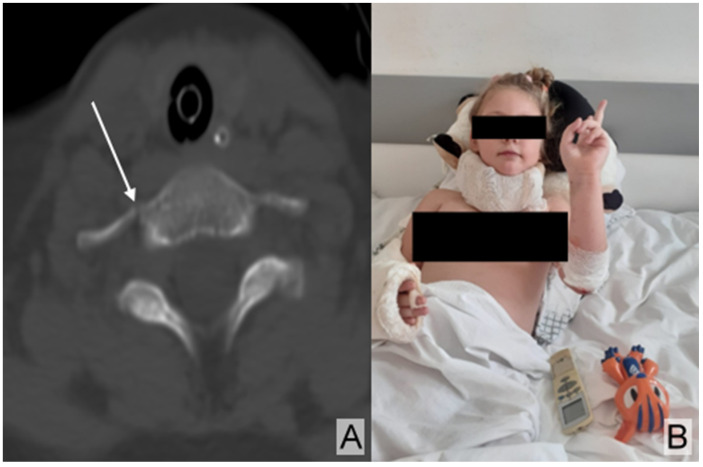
(A) Spine CT showing a fracture of the transverse process (arrow) of the seventh cervical vertebra with minimal displacement and no other detectable fracture lines in the cervical spine. (B) Patient immobilized in a cervical collar.
